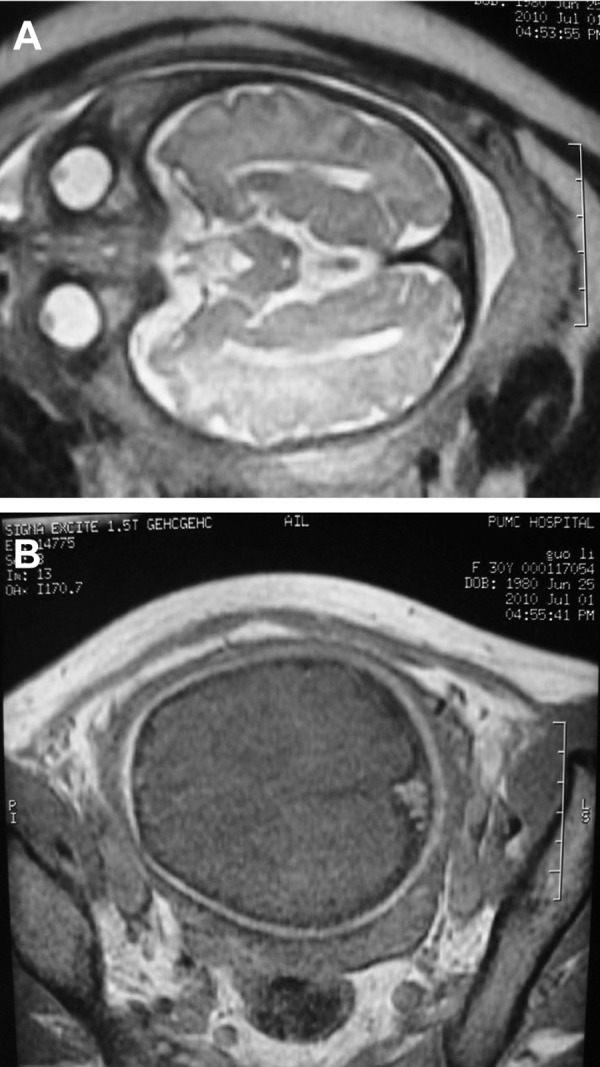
MRI images of the fetal head at 39 weeks’s gestation shows the torcular thrombosis becomes smaller than before. A. T2 weighted images at axial plane, B. T1 weighted image at axial plane.
